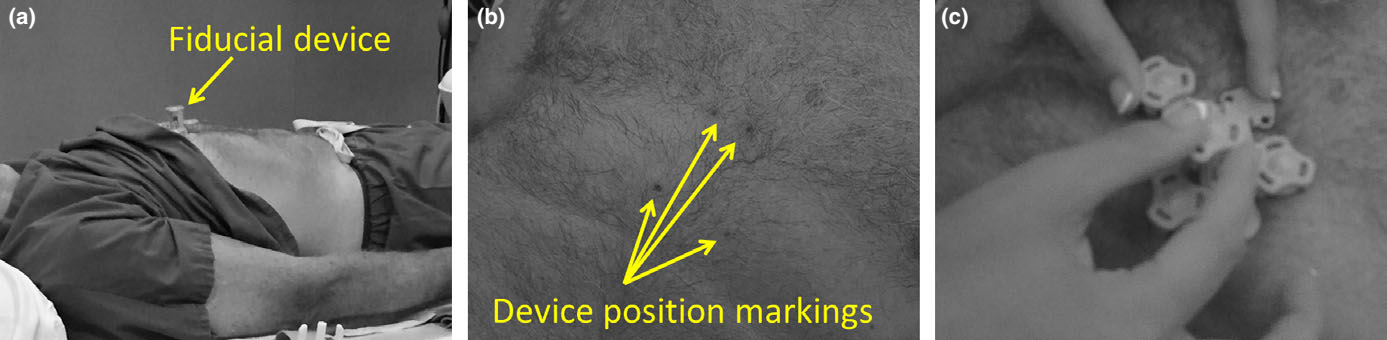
(a) Photo of the prototype fiducial device place on the volunteer. (b) Photo of the ink markings indicating the device position during MRI. (c) Photo of the replacement of the device for US imaging.
